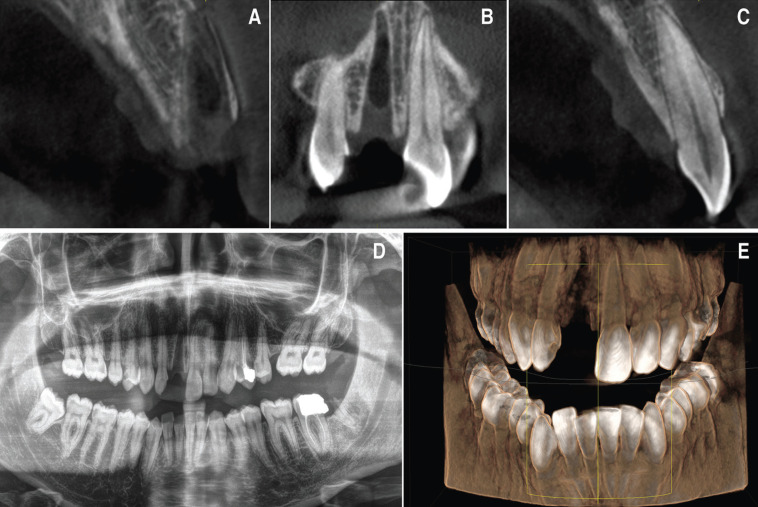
Pre-treatment radiographic study. A) Axial tomographic cut of the socket after the avulsion of the right upper incisor. B) Coronal view of the socket after the avulsion. C) Axial tomographic cut of the upper left incisor. D) Initial orthopantomography after tooth avulsion. E) 3D reconstruction of the patient’s bone jaws and teeth following dental trauma.
